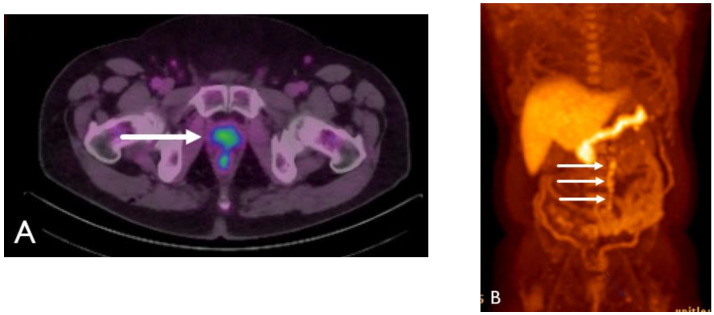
Fifty-six-year-old male with prostate cancer, status post-combination radiation and hormone therapy, now presenting with rising PSA. (A) Axumin-fused PET/CT image shows Axumin uptake within the prostate gland (arrow) concerning for local recurrence. MIP image (B) shows Axumin uptake within a chain of left peri-aortic lymph nodes (arrows) extending to the level of the kidneys.
